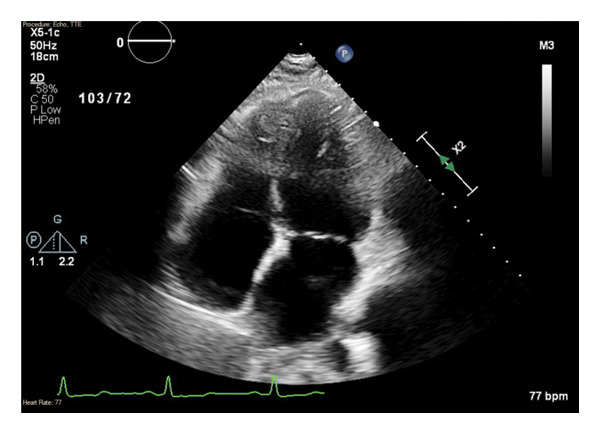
Echocardiogram on hospital presentation from the apical 4‐chamber view, demonstrating normal structure and function.
